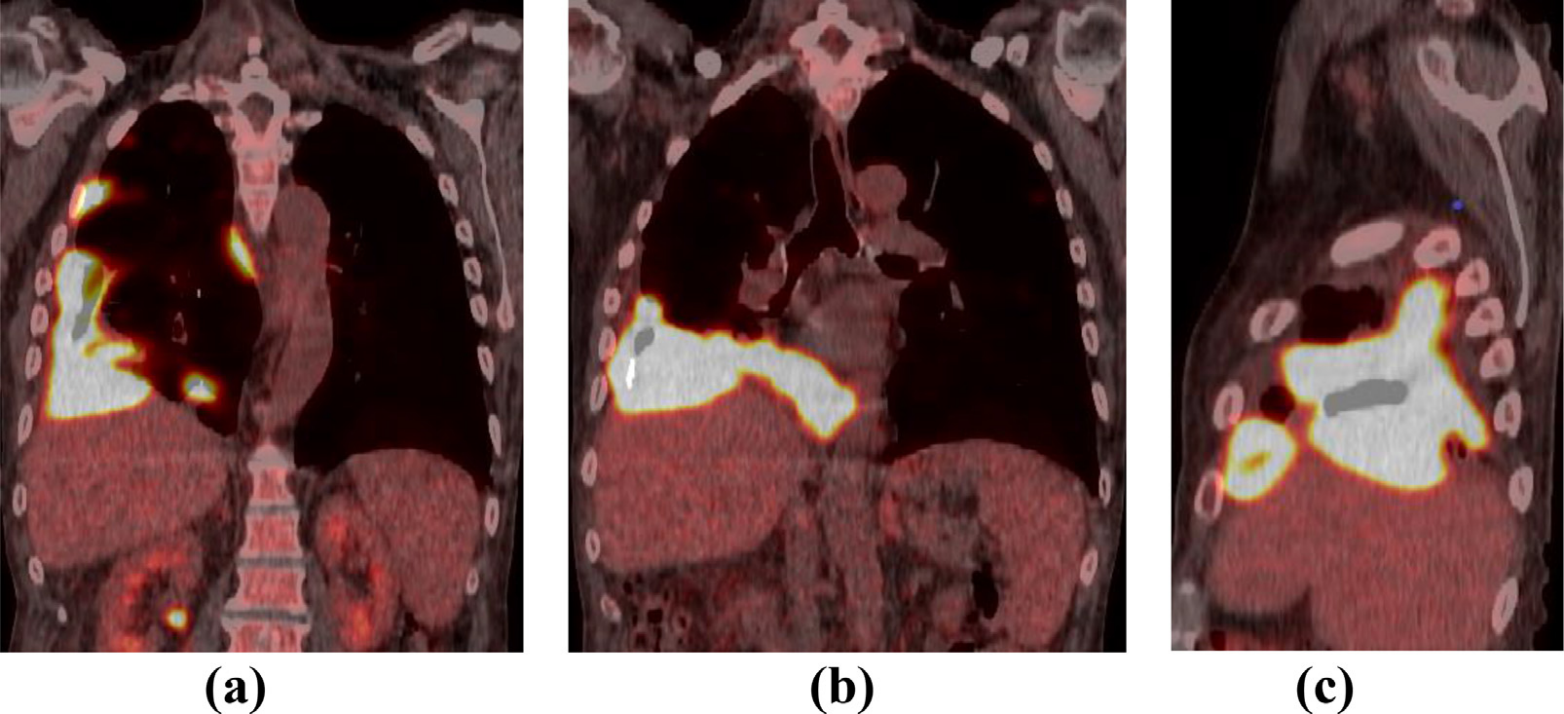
PET CT images in coronal and sagittal planes showing extensive involvement of the right lung and pleura.
